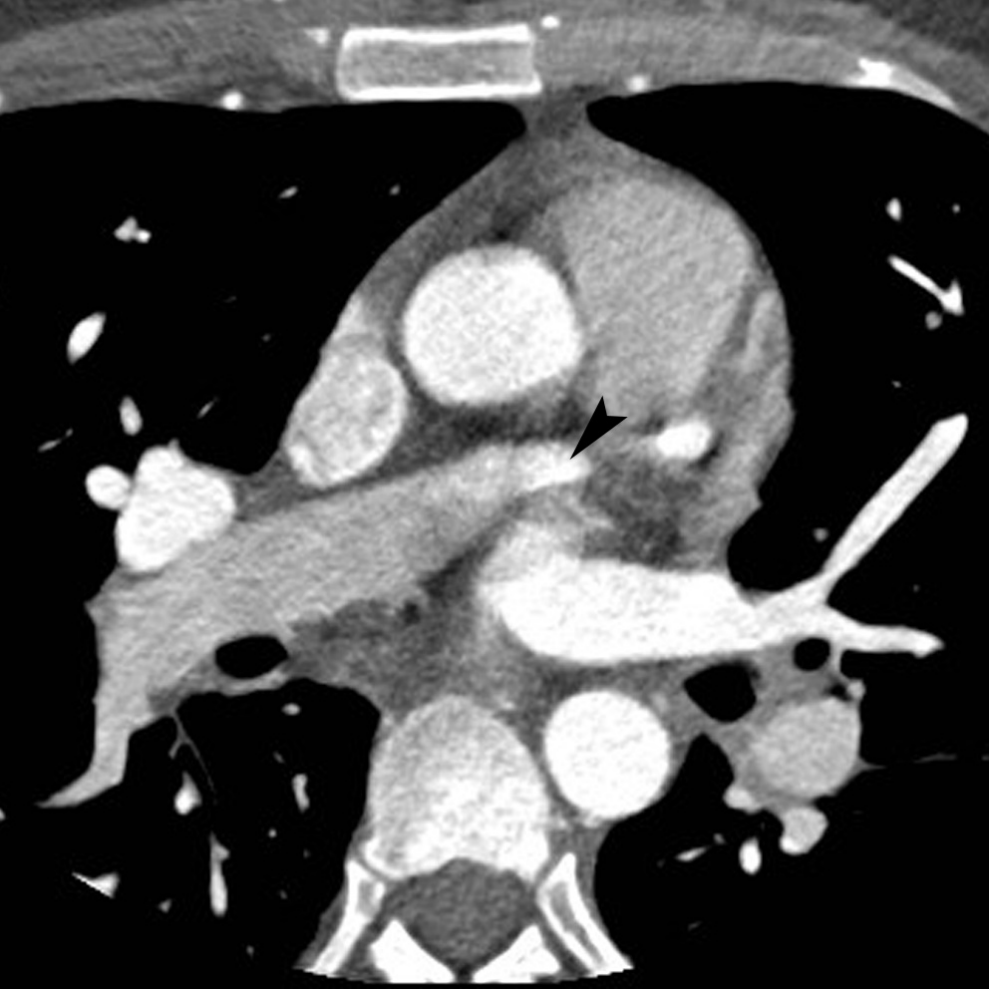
Anomalous LCX artery from RPA Axial coronary CT angiogram image demonstrating the anomalous origin of the left circumflex artery (arrowhead) from the right pulmonary artery. Note the retrograde opacification of the right pulmonary artery.
